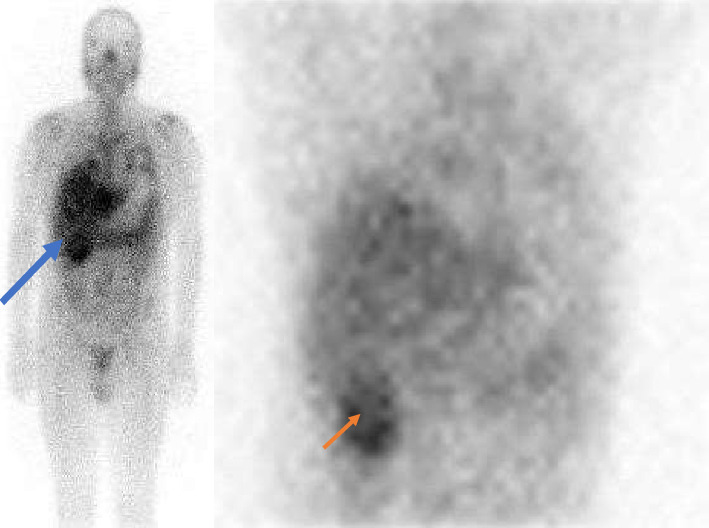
Increased focal uptake in the lungs corresponding to cavitary lesion on CT scan (blue arrow) uptake in the right upper quadrant corresponding to the perinephric collection (orange arrow).
